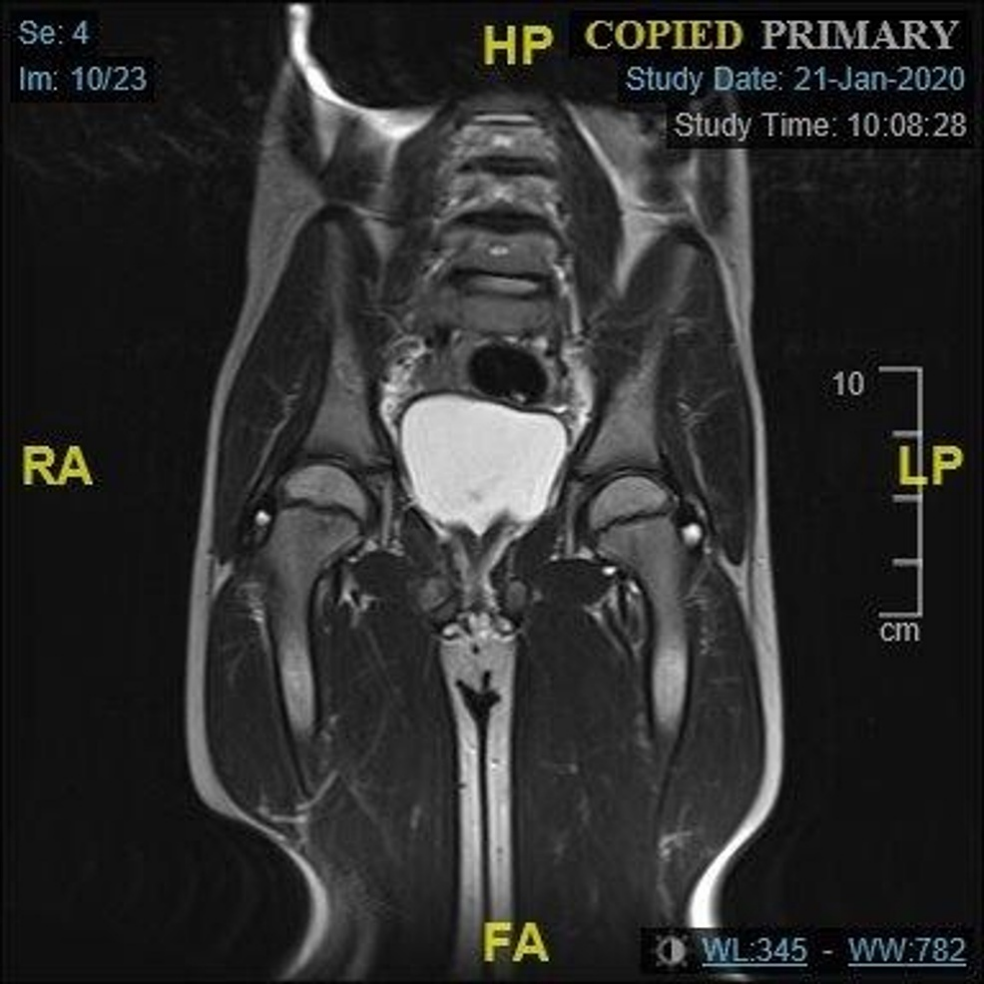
Coronal sections of T2-weighted MRI demonstrating almost complete resolution of the abnormal high signal in the right obturator internus and pectineus, and interval improvements in the inferior aspect of the left sacral ala.
